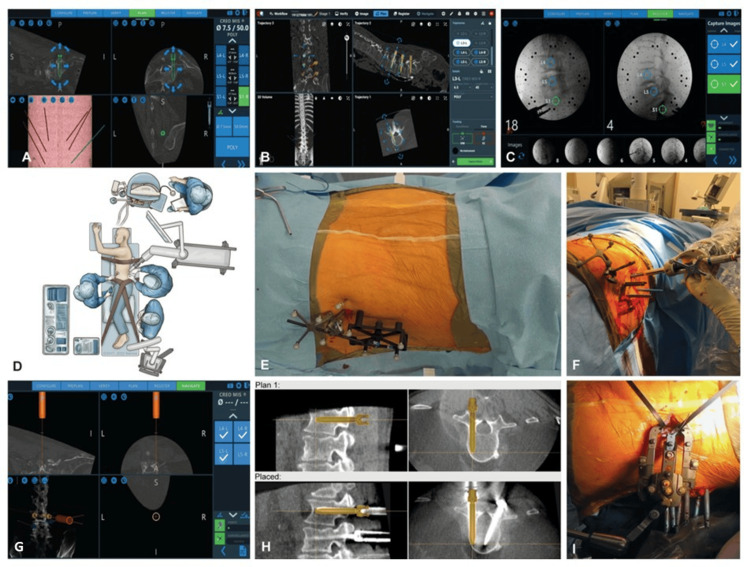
Screw planning, operating room set-up, and surgical procedures. (A-C) CT images are imported into screw planning software preoperatively, and placements are planned. (A) Screw plans (right S1) in the axial, coronal, and sagittal planes. Arrows along the screw allow fine adjustments. Image on the lower left shows trajectories of the robot arm; on the upper right, the estimation of screw size is shown. (B) Sagittal and coronal views of the entire screw trajectory plan. Vertebral levels are labelled, decreasing the risk of wrong-level surgery. (C) Fluoroscopic images used for registration are merged with preoperative CT images. Each level is registered separately. (D) Operating room set-up. The patient is positioned in the lateral decubitus position on the Jackson table with the left side up. The primary surgeon stands on the right side of the table during the entire procedure. The patient’s upper thorax and hips are taped to the table. The tape is crossed bilaterally over the left hip to stabilize the iliac crest, pull it down, and widen the working corridor. (E-I) Surgical procedure. (E) A dynamic reference base array and surveillance marker are placed on the posterior superior iliac spine and iliac crest, respectively, to aid with robot-assisted navigation (top view). (F) Robot arm position over the planned entry points (lateral view). (G) Simultaneous view of software, showing real-time orientation of the robot arm with respect to vertebrae. Notably, the trajectory is more vertical on the right side than on the left. (H) Sagittal and axial views of the planned trajectory of the right L1 vertebral body pedicle screw (top row). Post-procedure, sagittal and axial views of the actual placement of the pedicle screw of the right L1 vertebral body compared with the planned trajectory (golden overlay), showing the offset (bottom row). (I) Minimally invasive approach for interbody fusion (top view). Screw towers are left in place while performing interbody fusion. Used with permission from Barrow Neurological Institute, Phoenix, Arizona
